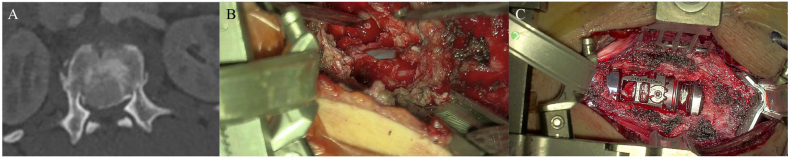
(A) Axial CT scan showing an L1 vertebral body fracture with a large endocanalar fragment. (B) Exoscopic intraoperative view demonstrating complete dural decompression (patient in right lateral decubitus, surgical approach from the left). The exoscope provided magnified, high-definition visualization with improved depth perception, facilitating safe decompression. (C) Exoscopic view of the cage in final position. The patient was then positioned supine for percutaneous fixation.
